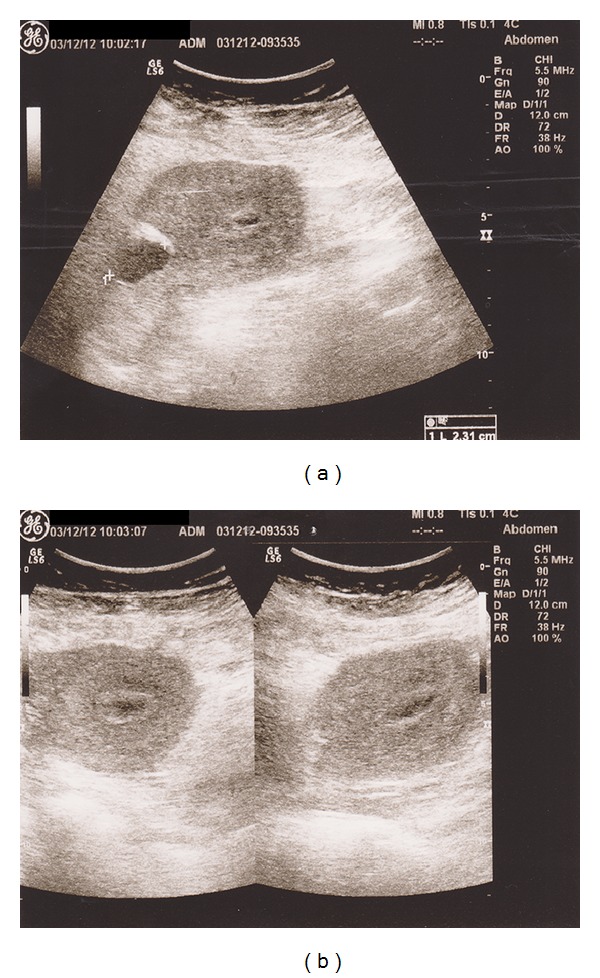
A lesion, 20 mm in diameter, consistent with fluid collection, was detected on gray scale sonography.
